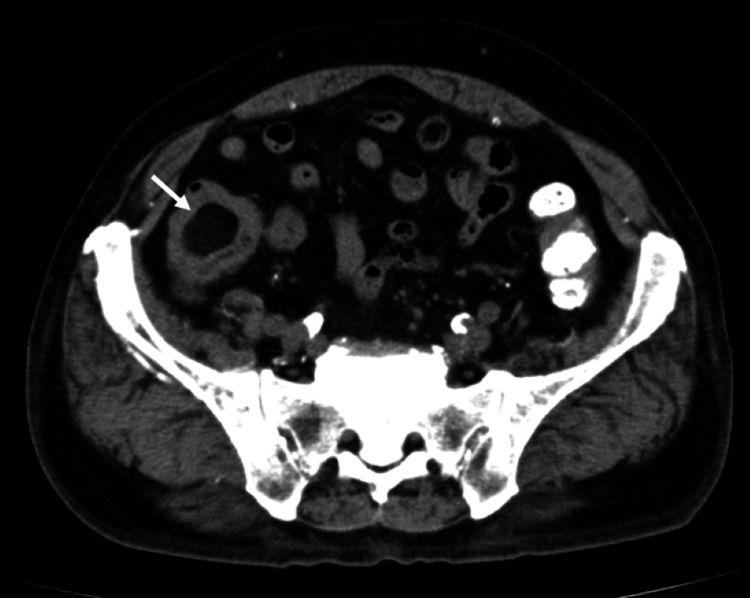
Plain abdominal computed tomography (CT) A low-density mass lesion was identified in the cecum (white arrow), suggestive of a lipoma.
